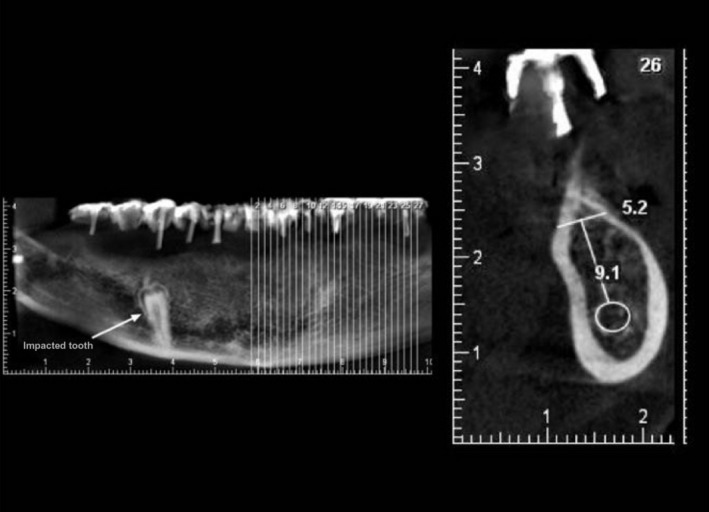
CBCT scan of alveolar ridge with radiographic stent
